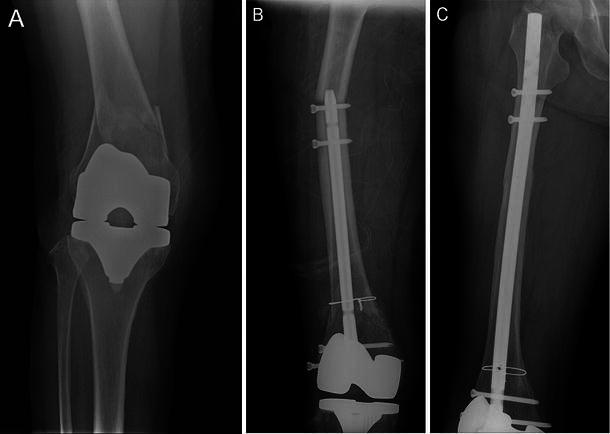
a Case 2. Preoperative X-rays of a periprosthetic fracture of the *right* knee in a 62-year-old woman. b A radiograph of refracture at the tip of the retrograde nail taken 3 months later after the RISN fixation. c Follow-up radiograph taken 2.5 years later after fracture fixation with a femoral intramedullary nail showing callus formation
